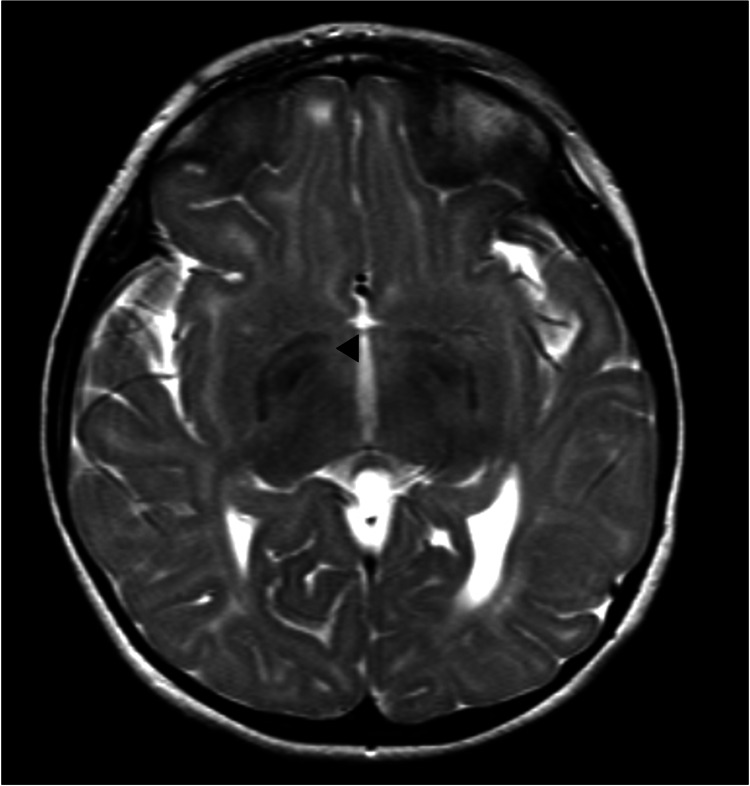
Axial T2 Weighted Imaging The T2 hyperintense signal within the medial medullary lamina appears more well-defined on follow-up MRI flanked by hypointense signal within the medial and lateral segments of the globus pallidus (arrowhead).
